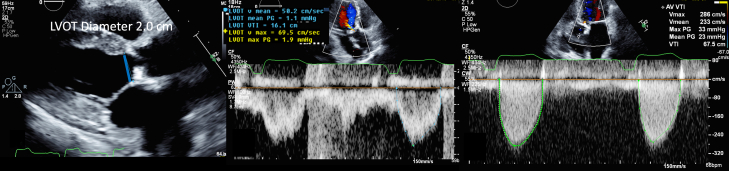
Calculated Aortic Valve Area by the Continuity Equation Aortic valve area is calculated by the continuity equation using the left ventricular outflow tract (LVOT) diameter from the parasternal long-axis view, the LVOT velocity, and aortic valve velocity.
